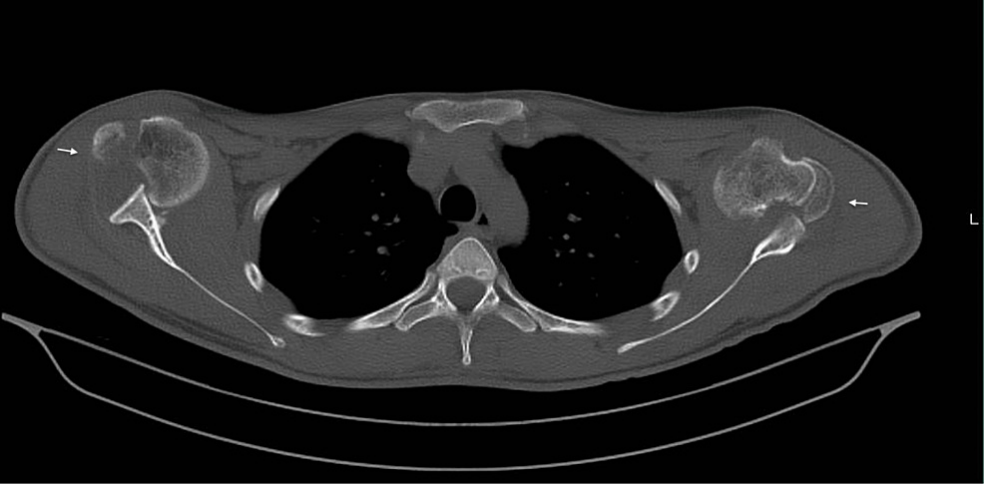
CT scan showing bilateral anterior shoulder dislocation with proximal humerus fractures and Hill-Sachs lesions CT, computed tomography.
